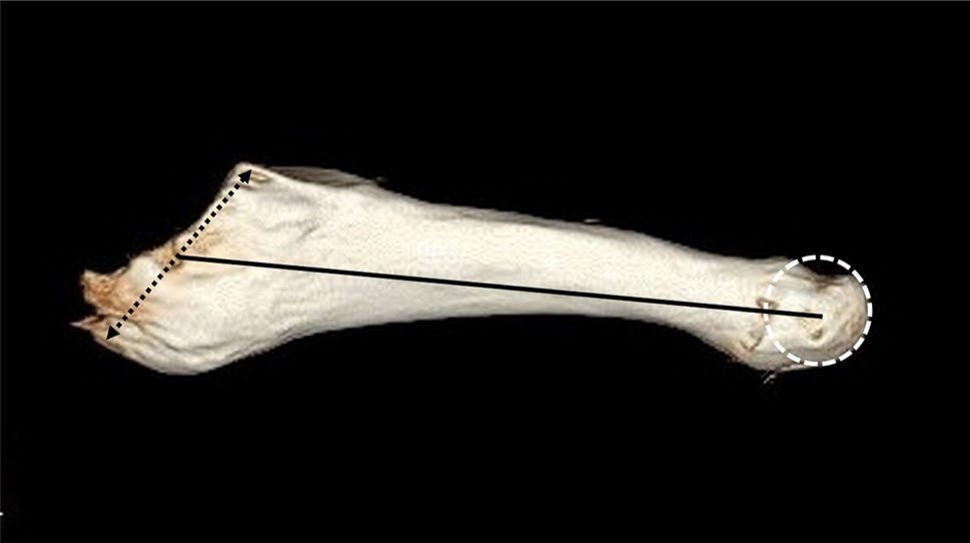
Computed tomography 3D reconstruction image of the fifth metatarsal bone. The midpoint of the metatarsal’s oblique base (black double-headed arrow) was marked as the proximal reference point for length measurement. The midpoint of the fifth metatarsal head transverse diameter (white dotted circle) was defined as the distal reference point for length measurement. The measured metatarsal length (FML) is depicted as the black line
